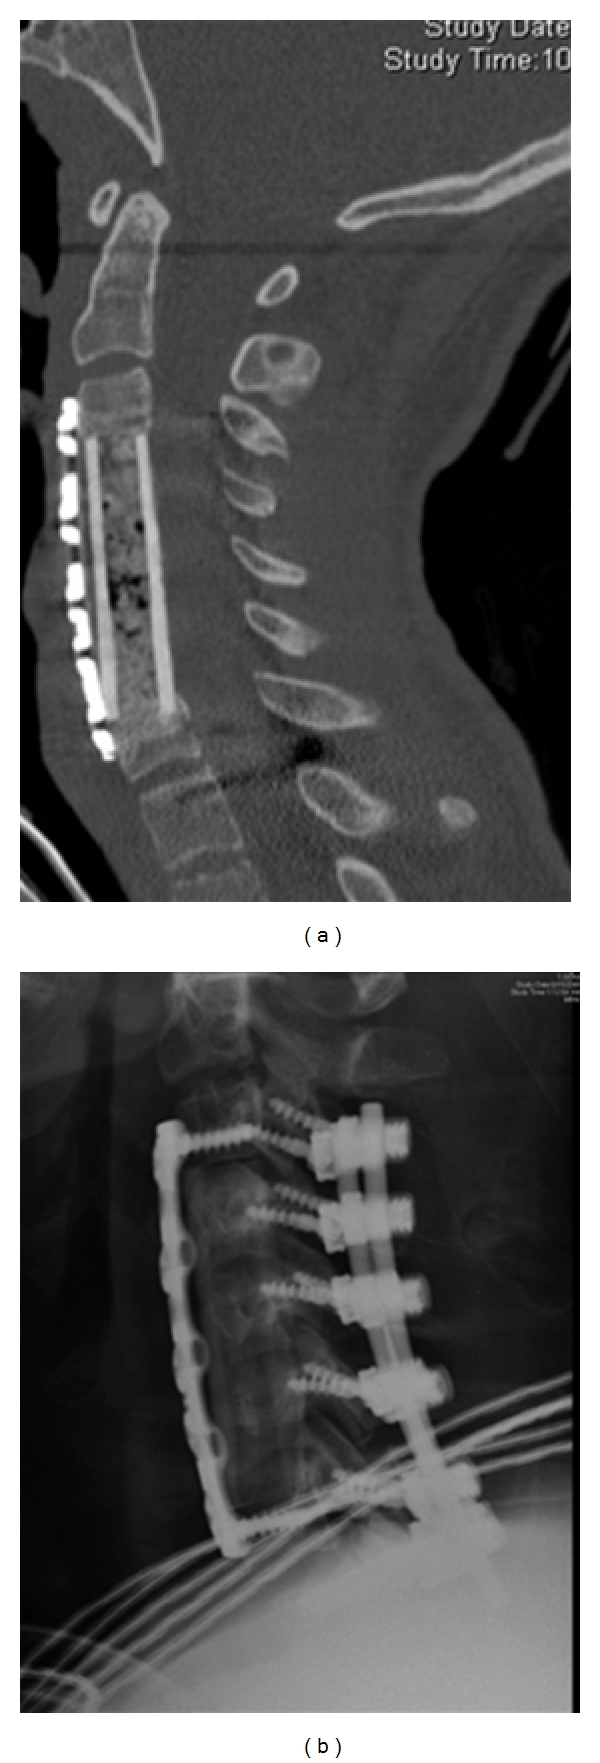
(a) Sagittal reconstructed CT scan of patient who underwent 3-level corpectomy. (b) Lateral radiograph of same patient. Posterior fusion was performed to increase stability of the construct.
